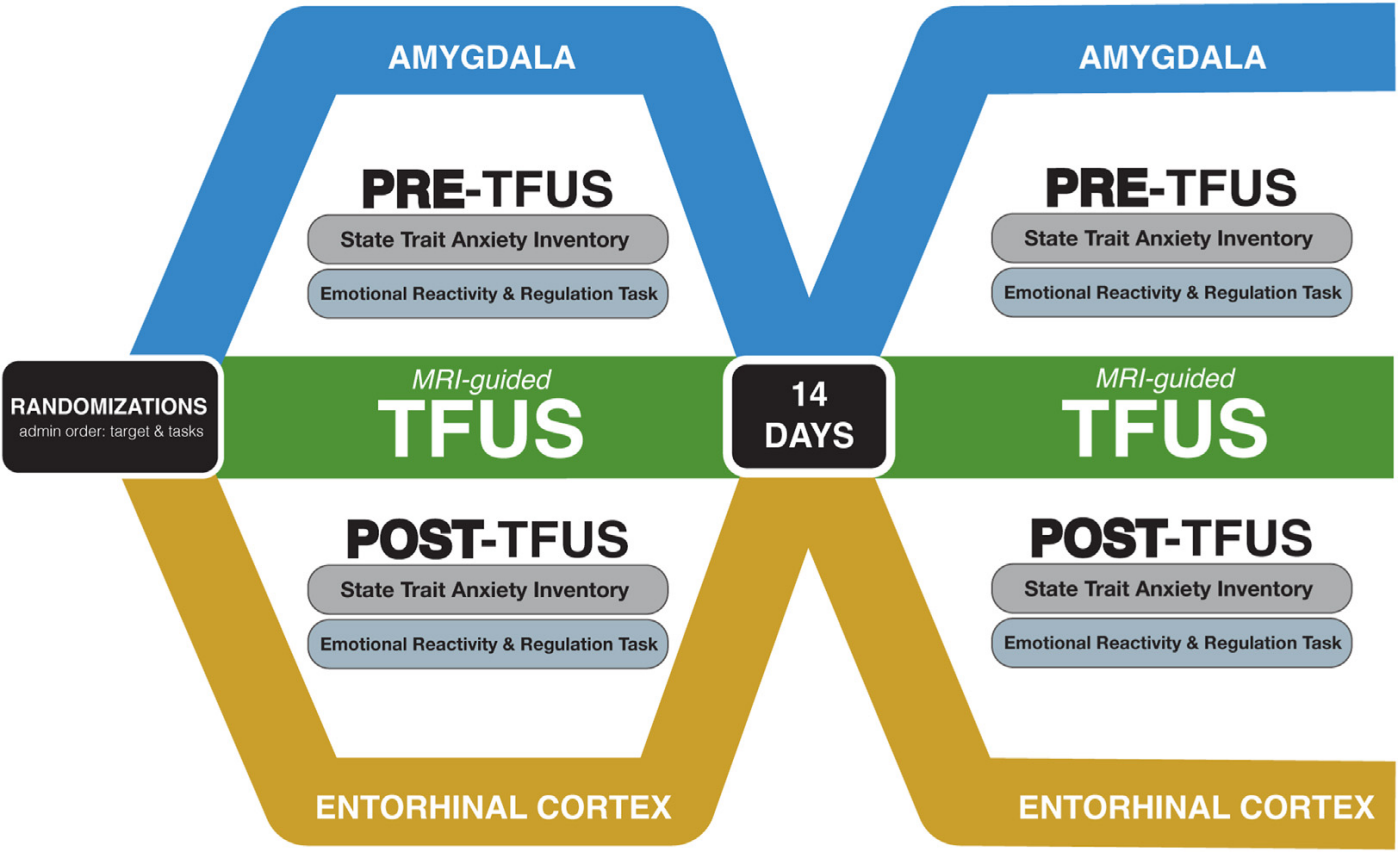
Study design. A visual representation of the randomized, double-blinded, within-participant crossover study design. Participants completed 2 study visits separated by 14 days targeting either the right amygdala or left entorhinal cortex. Examples of amygdala and entorhinal cortex transcranial focused ultrasound (TFUS) targeting are provided in Figure 2, and a chart detailing sample demographics is provided in Table 1. MRI, magnetic resonance imaging.
